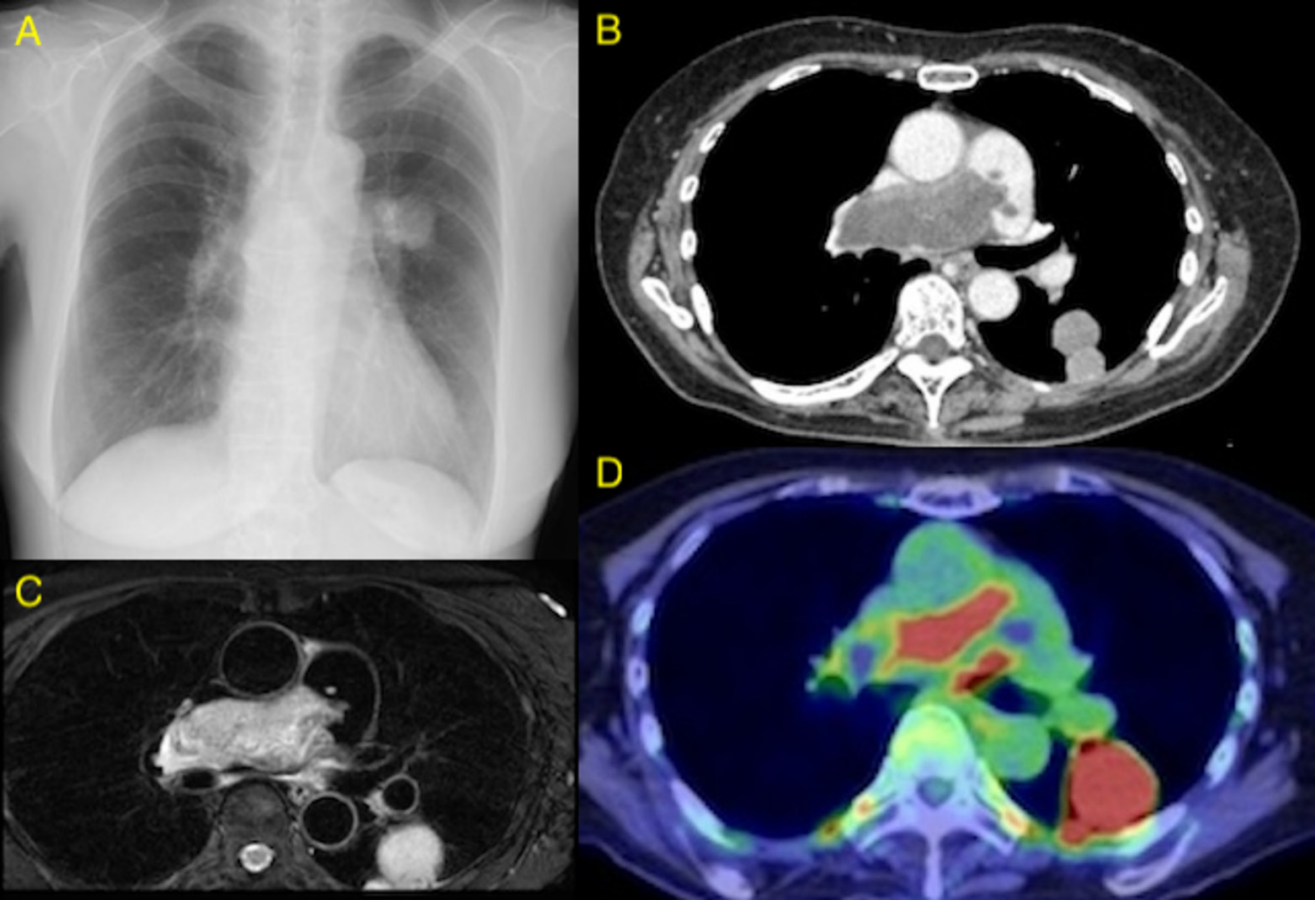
(A) Chest radiograph showing a mass shadow in the left middle lung field. (B) Contrast‐enhanced computed tomography showing a contrast defect occupying the entire lumen of the main pulmonary artery and a mass shadow in the left lower lobe. (C) Contrast‐enhanced magnetic resonance imaging showing a mass lesion in the main pulmonary artery, characterised by high signal intensity on T2‐weighted images with internal contrast enhancement. (D) Positron emission tomography showing standardised uptake value (SUV) max = 13.6 in the main pulmonary artery with areas of poor uptake within the lesion.
